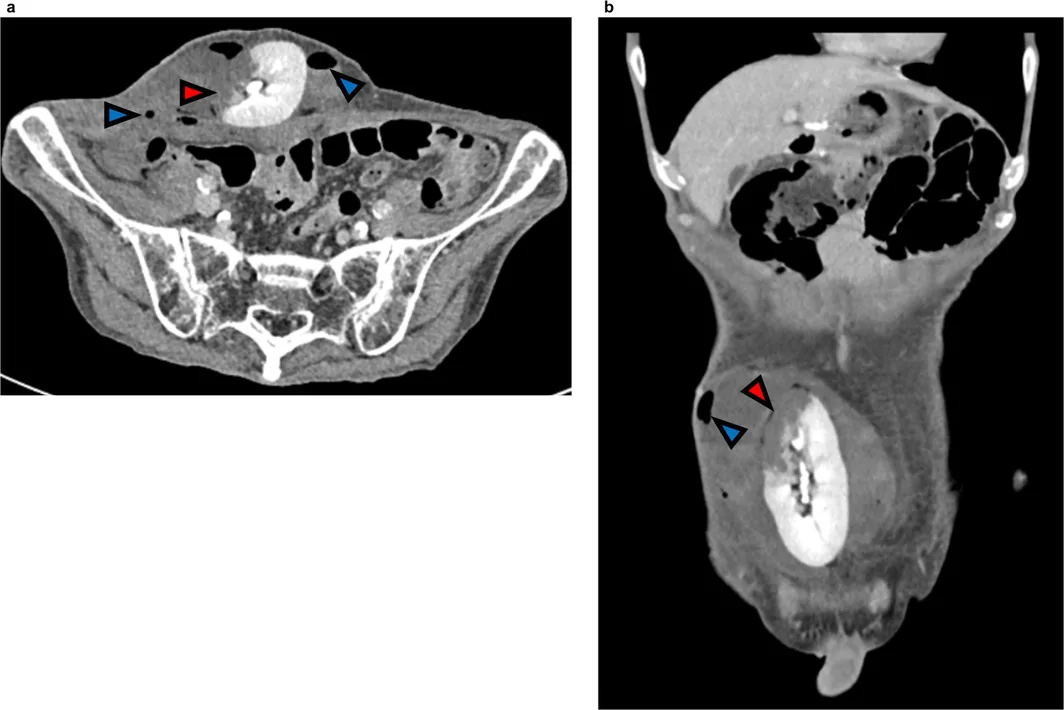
Contrast‐enhanced computed tomography at presentation. (a) Axial image showing the transplanted kidney located in the subcutaneous tissue of the right lower abdomen. Blue arrowheads indicate perirenal gas, and the red arrowhead indicates a partially non‐enhancing ischemic area within the renal parenchyma. (b) Coronal image demonstrating subcutaneous graft malposition with surrounding gas and fluid collection; blue arrowhead indicates gas, and the red arrowhead indicates a focal ischemic area.
